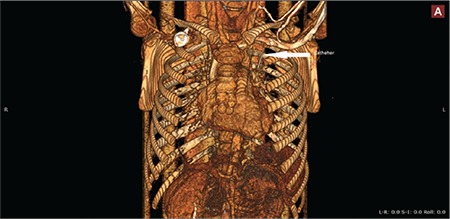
Thorax computed tomography angiography of the patient showing the embolization of the catheter to the left pulmonary artery.
